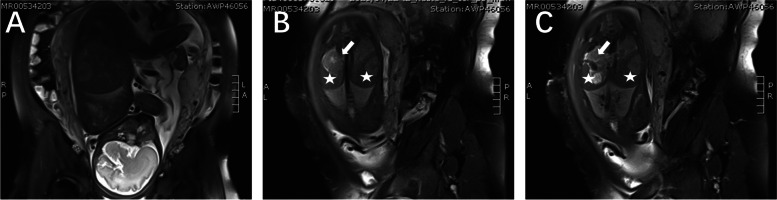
Prenatal MRI features of the fetal hepatic mass. A-C A large hepatic mass (arrow) with high signal intensity was seen in a sagittal T2-W image compared to the normal liver (star)
